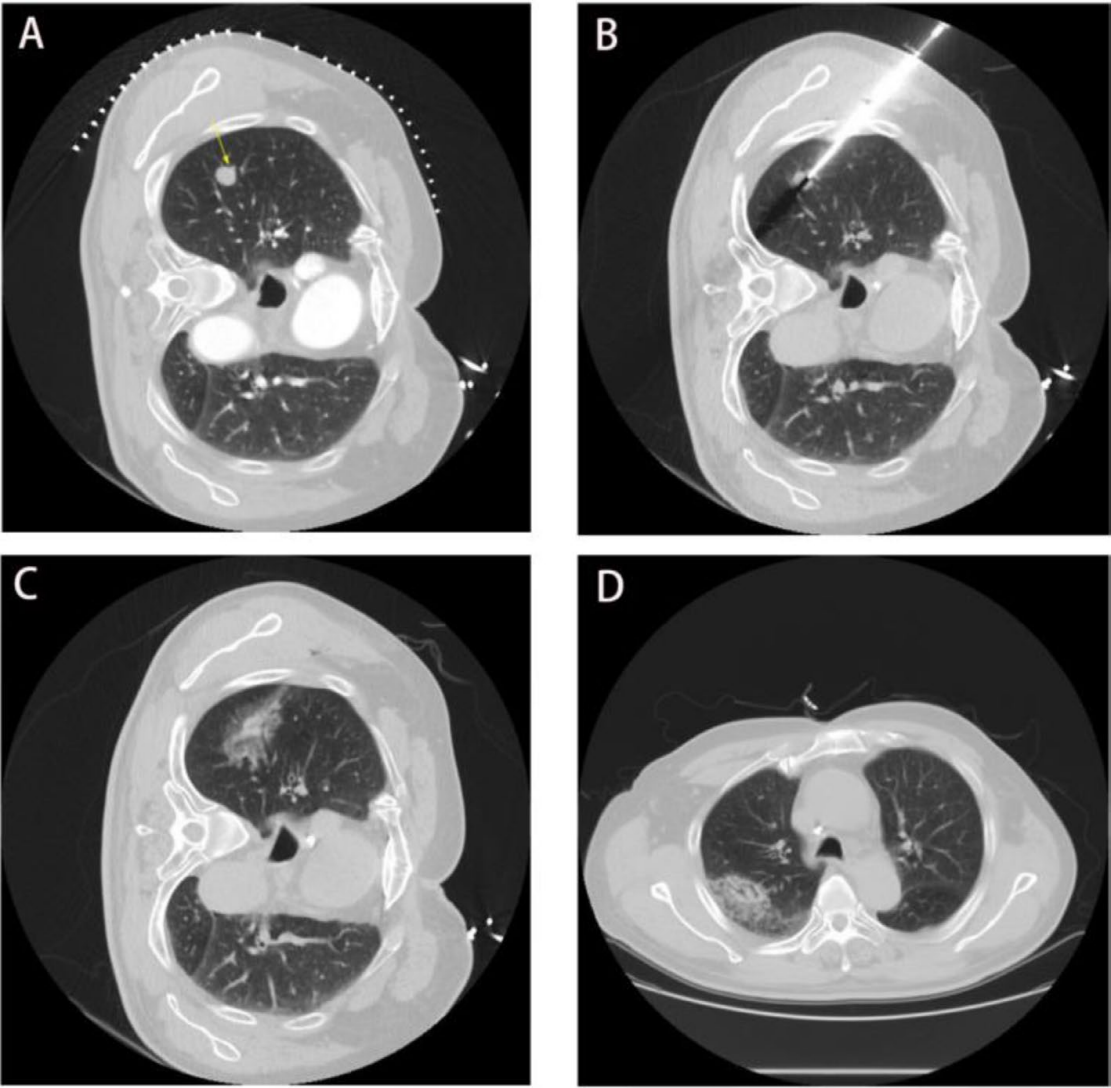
Typical case of microwave ablation procedure. (A) CT scan localization. (B) Ablation antenna in lesion. (C) Image at the end of microwave ablation. (D) Next‐day after microwave ablation.
